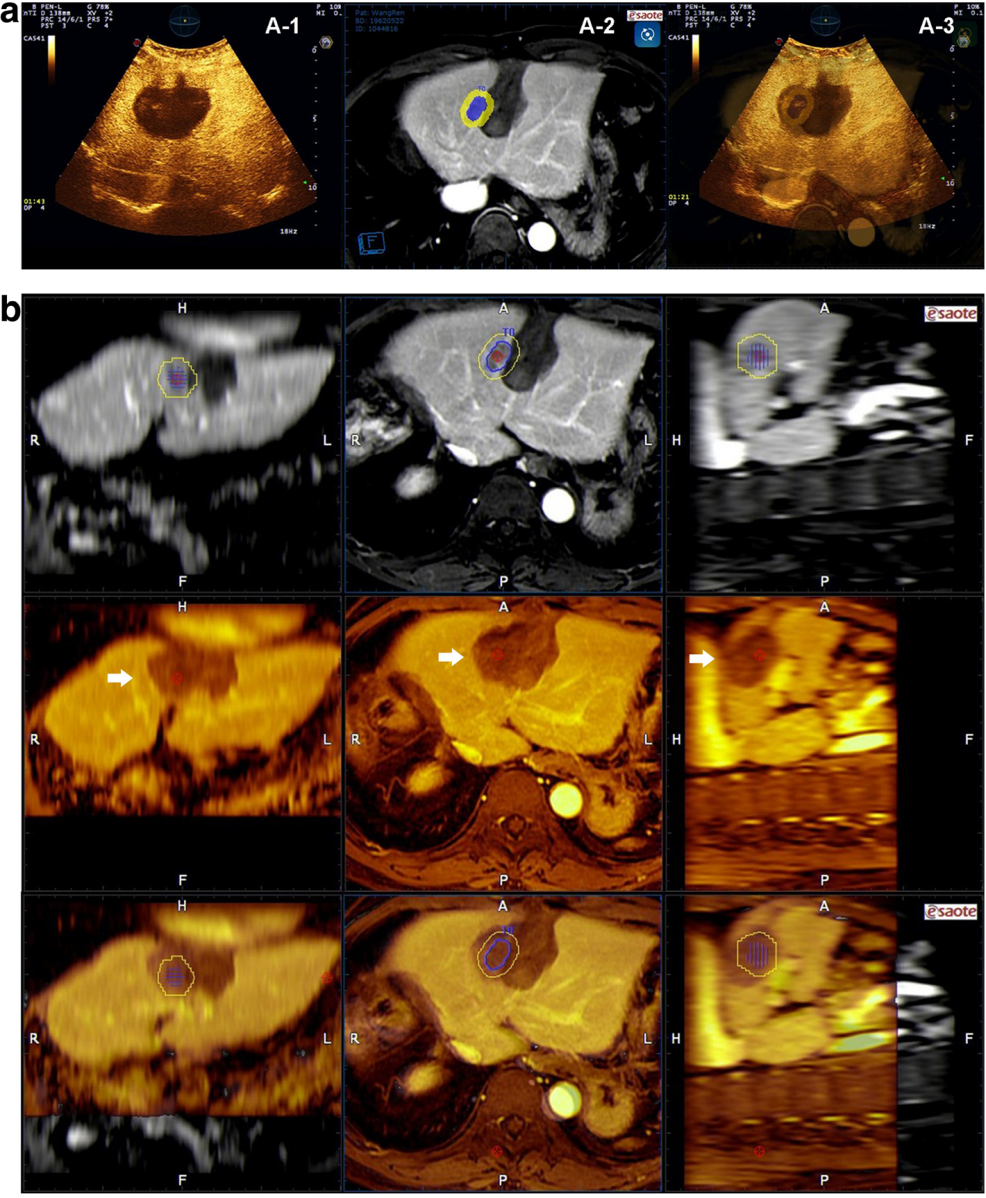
Medical images of case 1 in the clinical study. (A-1) CUES image of the area of ablation (dark). (A-2). Preoperative MR image (tumor shown in blue, and 5-mm AM shown in yellow). (A-3) CEUS-MR fusion image. The tumor and 5-mm AM were fully encompassed by the area of ablation. b MR-MR fusion image of case 1. *Upper panel*: preoperative MR images (tumor shown in blue, and 5-mm AM shown in yellow). *Middle panel*: postoperative MR images (arrow, ablation area shown in dark color). *Lower panel*: MR-MR fusion images showing that the tumor and the 5-mm AM were fully encompassed by the area of ablation
